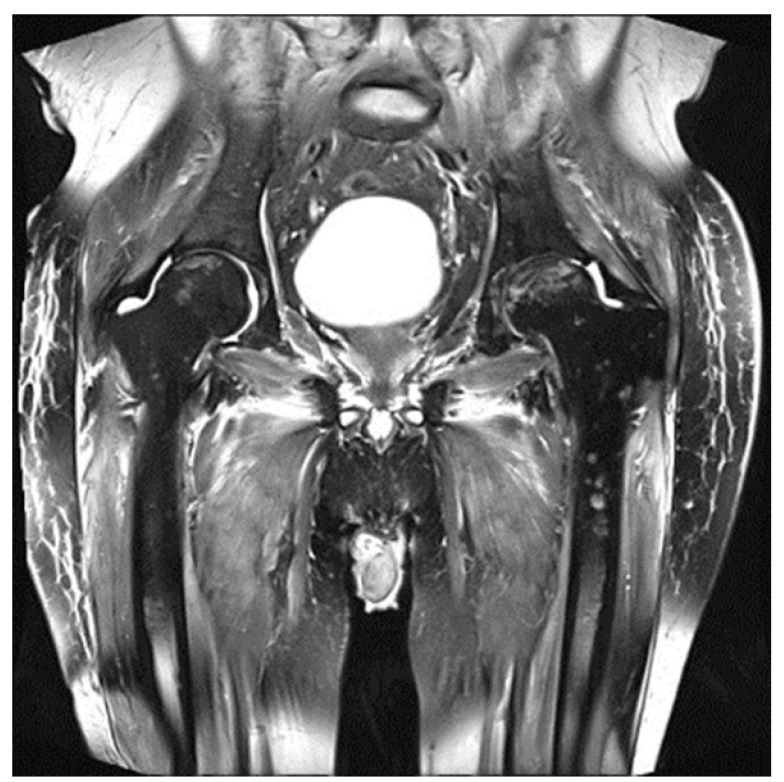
An emblematic case of bilateral femoral head osteonecrosis in a post-transplanted multiple myeloma (MM) patient. In the femoral head osteonecrosis is a double line sign with a low signal intensity and outer rim and high signal intensity inner line as demonstrated on the coronal T2-weighted image.
